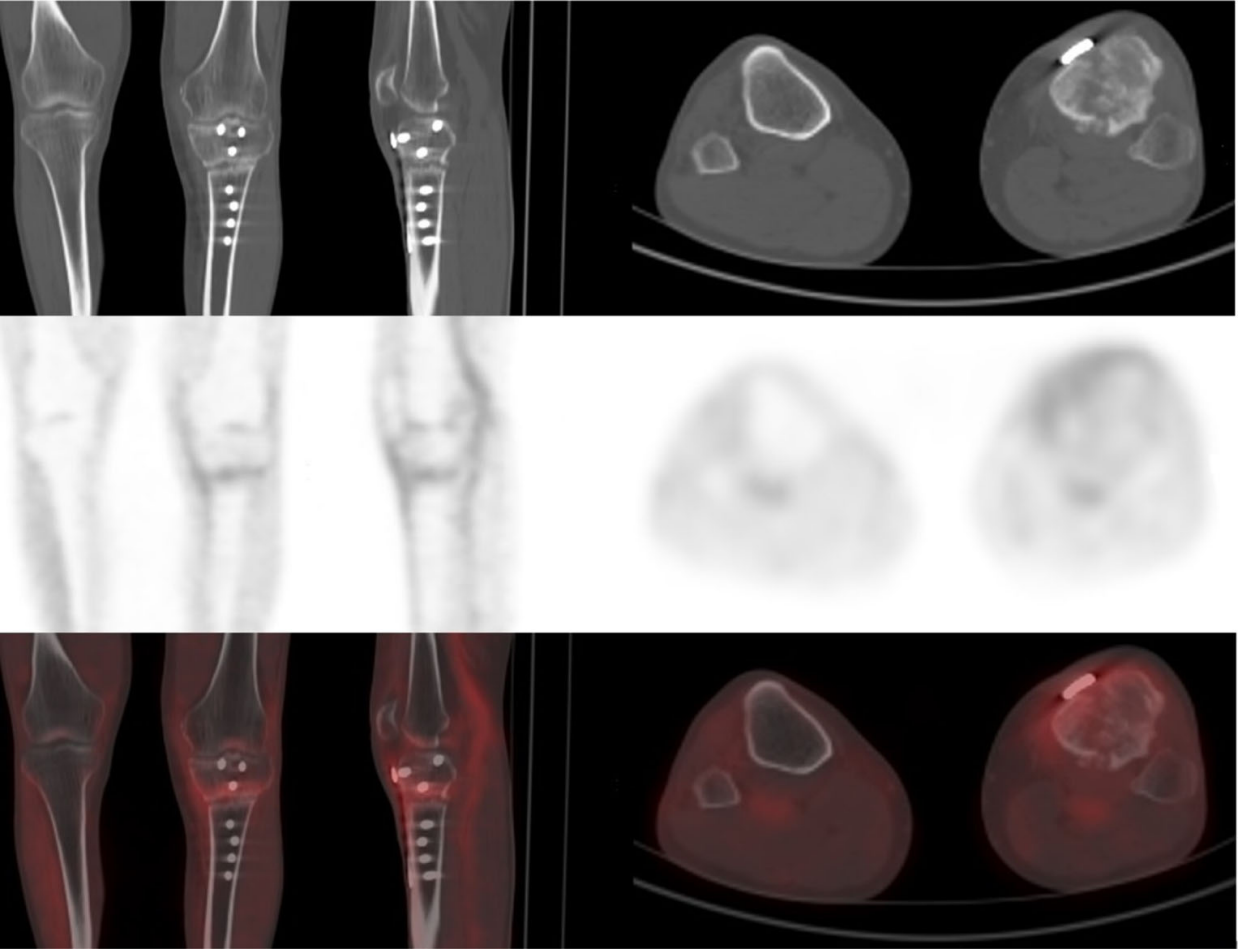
53-year-old patient with atrophic non-union fracture after a tibial wedge osteotomy. No complete osseous consolidation was seen for 9 months. [^18^F]FDG PET scan showed only slight, homogeneously distributed and not focally accentuated uptake (SUV_max_ 3.0, SUV ratio 1.8) along the fracture gap, which was fixed by a plate. The uptake was rated positive for infection in the first set of visual analysis (false positive). The SUV-based analysis, the second set of visual analysis, and the combination of both were rated negative for infection (true negative). In the follow-up, no infection was observed
